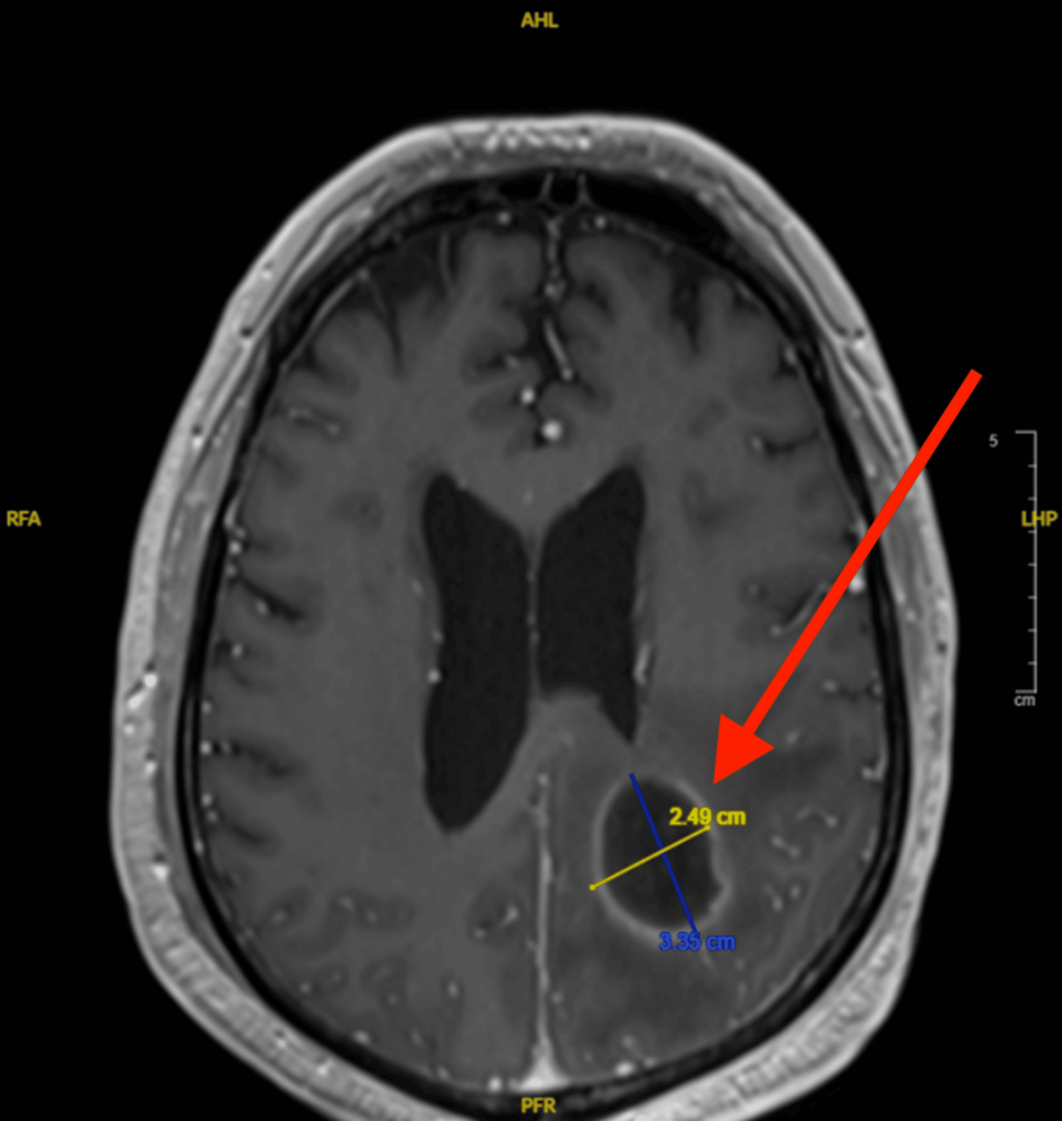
Contrast enhanced 3-D axial T1-weighted magnetization prepared rapid gradient echo (MPRAGE) MPR image depicting a 2.5 x 3.4 cm left parietal periventricular abscess. MRI study dated March 13, 2024. MPR: Multiplanar reconstruction
